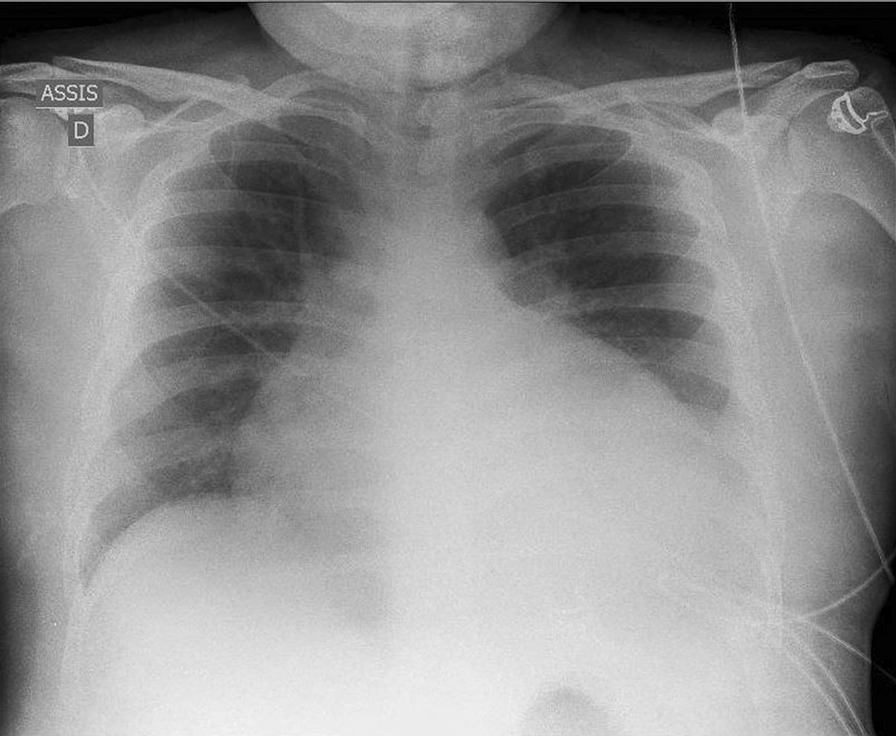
Chest x-ray shows cardiomegaly with bilateral hilar congestion
